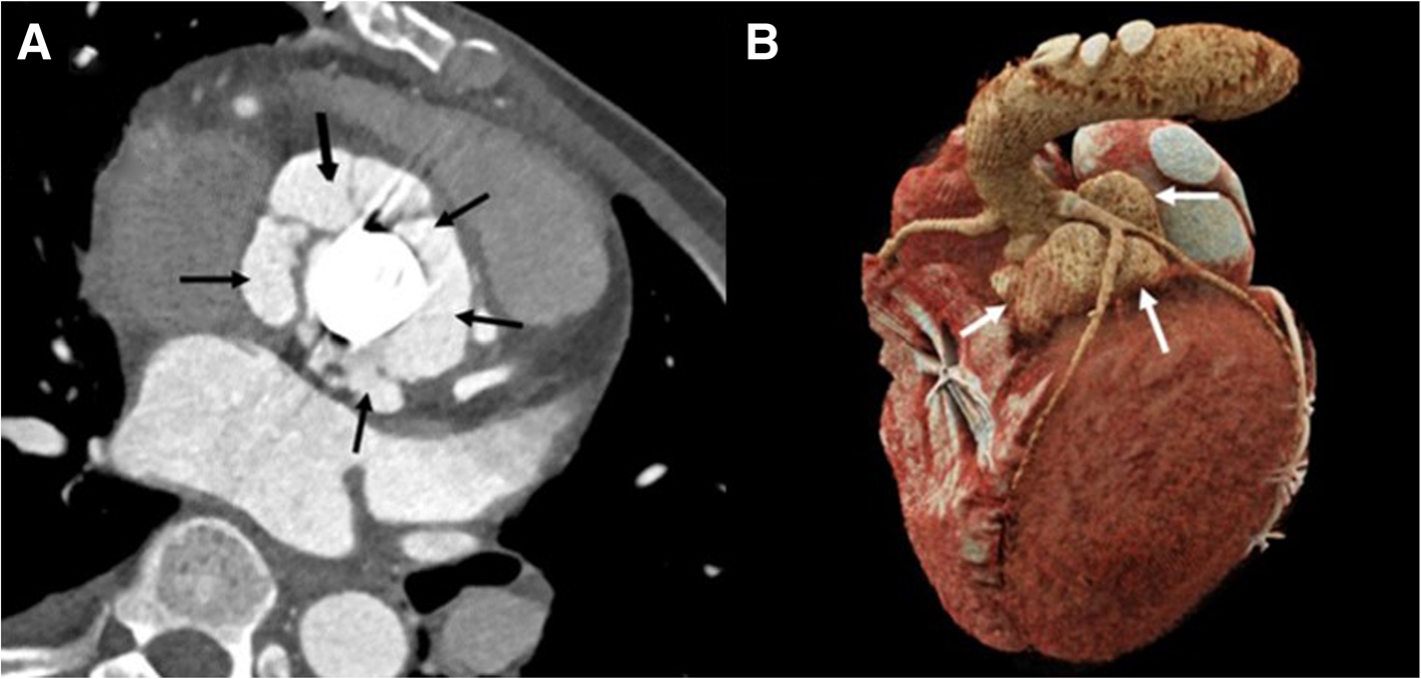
A 43-year-old man with a mechanical aortic valve. An aortic valve view (a) and cinematic reconstruction image (b) of cardiac CTA showing multiple paravalvular pseudoaneurysms (arrows). Noted the left anterior descending artery was located just above the pseudoaneurysm which demonstrated clearly by cardiac CTA. The pseudoaneurysm could not be detected on TTE due to the shadowing of the mechanical aortic valve
